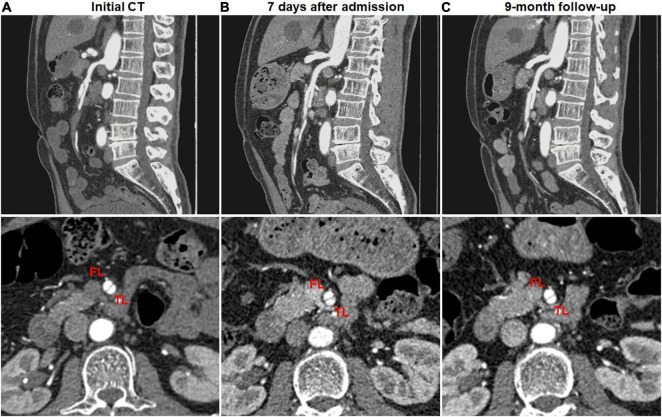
No change of type I spontaneous isolated superior mesenteric artery dissection on follow-up computed tomography (CT) angiography. (A) Initial CT angiography showed patent false lumen and true lumen with both entry and re-entry in a 53-year-old man. (B) One week after conservative management, type I dissection showed no interval change. (C) Nine month later, patent false lumen was sustained with both entry and re-entry as well as no aneurysmal dilatation.
